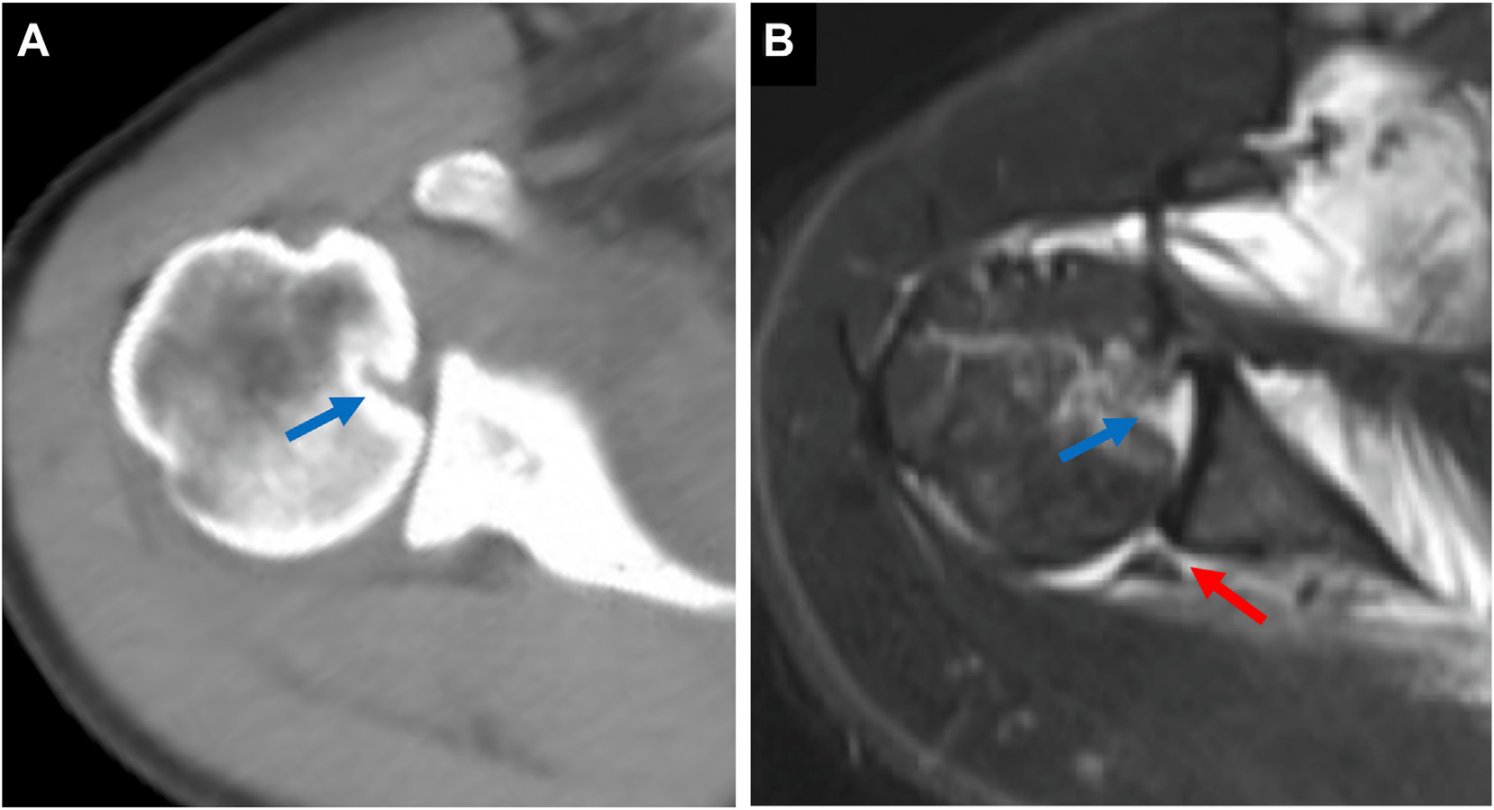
(A) CT and (B) T2-weighted fat-suppressed MRI images of Case 2, obtained at a local hospital, revealed a reverse Hill-Sachs lesion (*blue arrow*) and a posterior Bankart lesion (*red arrow*). *CT*, computed tomography; *MRI*, magnetic resonance imaging.
